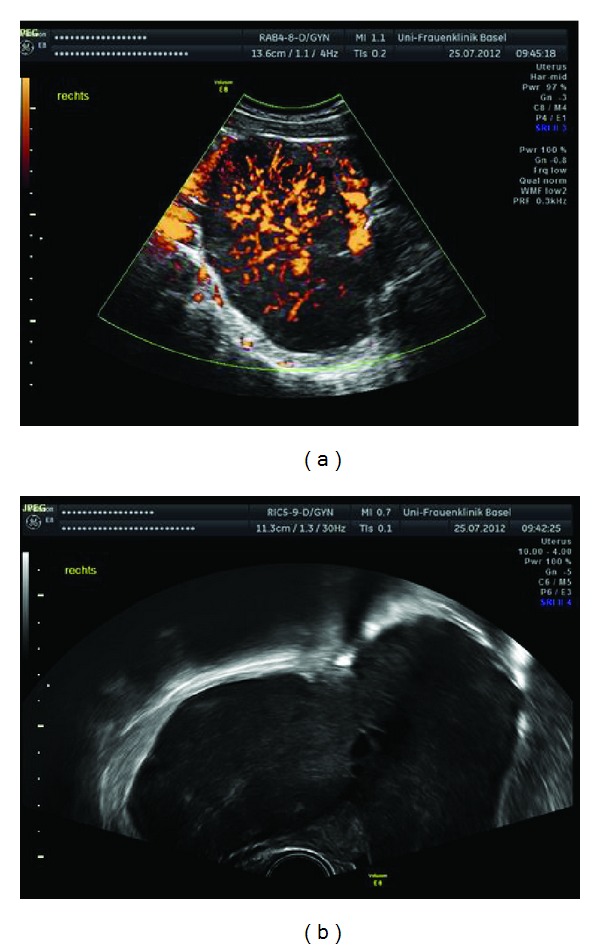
Initial ovarian ultrasound showed bilateral ovary masses.
